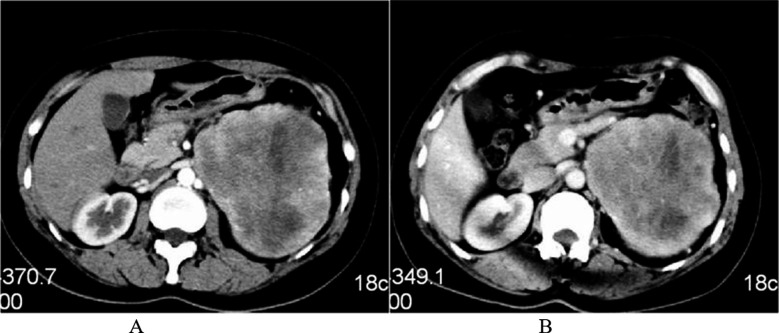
Enhanced CT manifestations of intra-abdominal DSRC. (A) There was moderate uneven enhancement in arterial phase, with no enhancement in necrotic and cystic areas; the CT value was 57 HU. (B) There was continuous mild uneven enhancement in portal venous phase; the CT was 63 HU. Invasion and metastasis.
